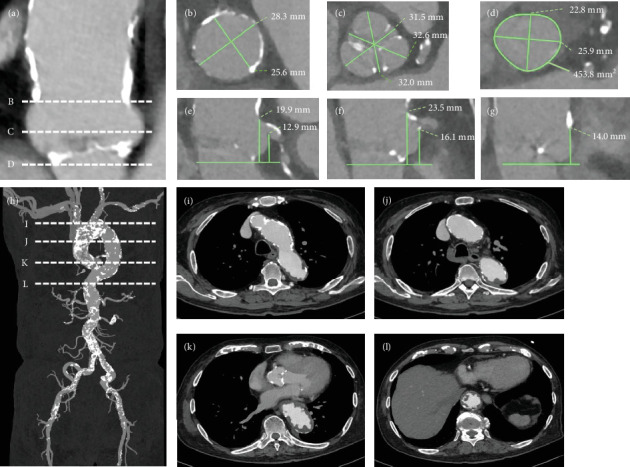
Multidetector CT of the aortic valve and access root. (a) Aortic valve complex, (b) aortic annulus, (c) valsalva, (d) sinotubular junction, (e) left coronary height and cusp height, (f) right coronary height and cusp height, (g) noncoronary cusp height, and (h–l) CT scans showing a shaggy and heavily calcified aorta from the aortic arch to the descending aorta. Abbreviation: CT, computed tomography.
